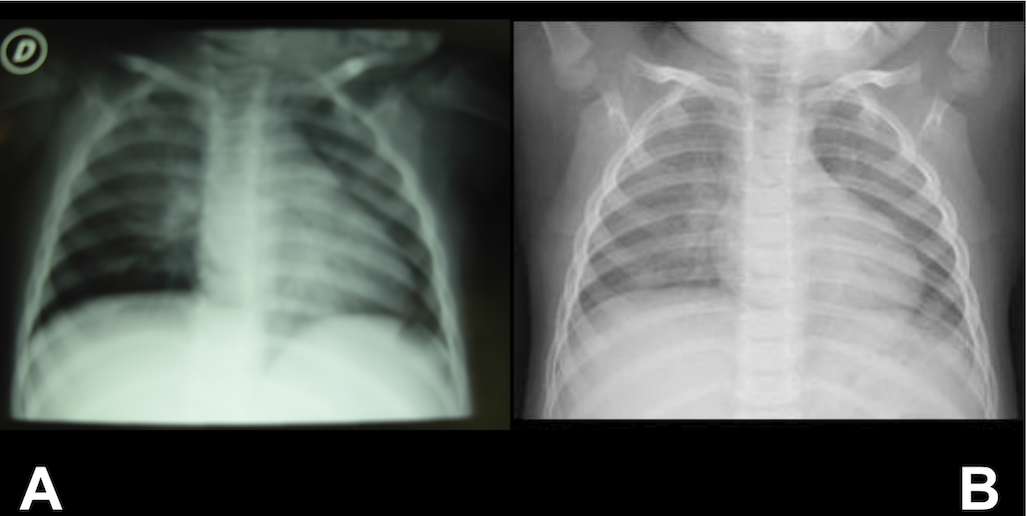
Chest x-ray on admission (*a*) and five days after (*b*).
